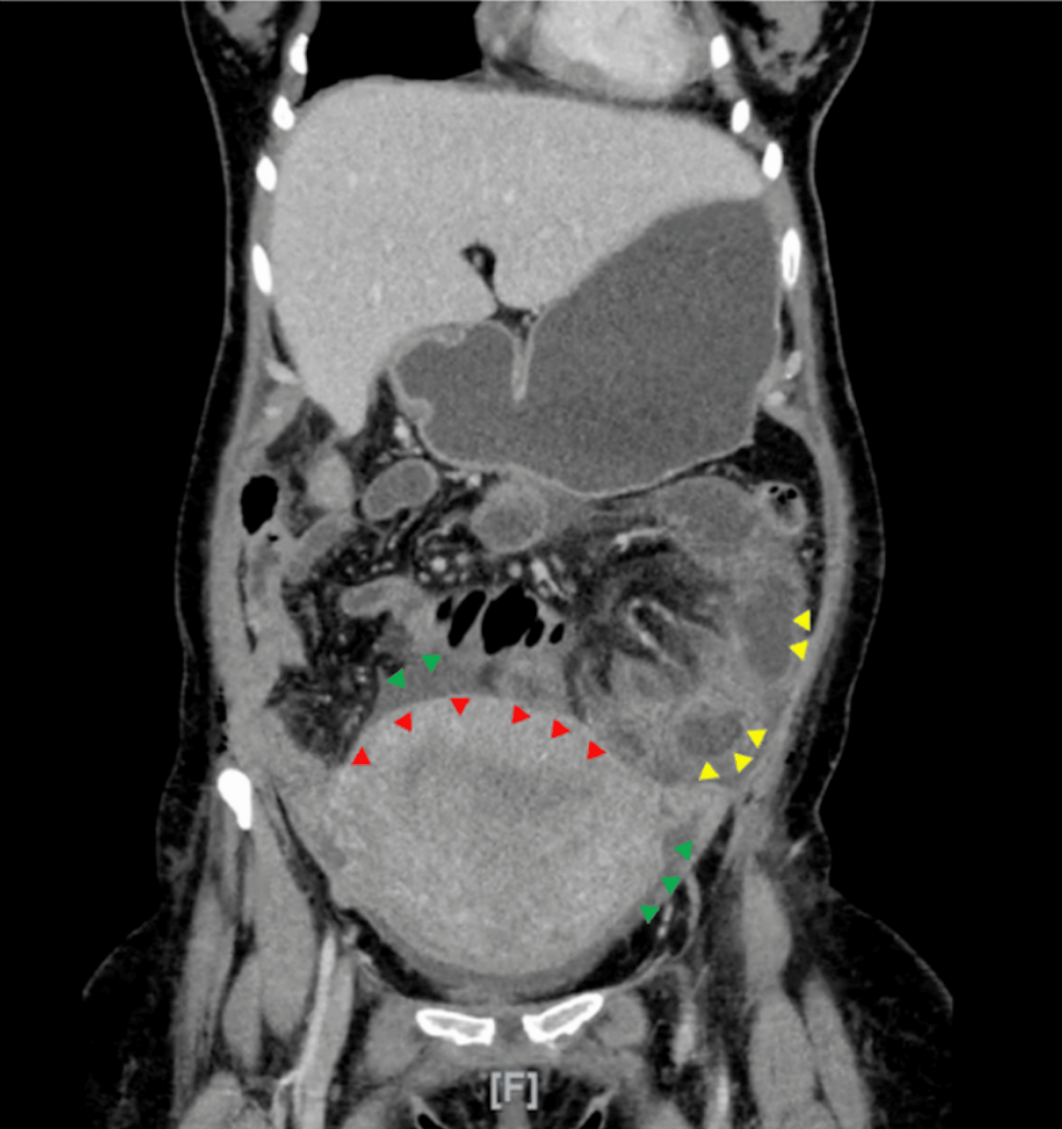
Coronal slice through contrast-enhanced CT abdomen and pelvis Performed on the day of readmission, corresponding to post-cesarean section day 4, showing red arrowheads, enlarged uterus consistent with the postpartum period; green arrowheads, pockets of free fluid in the pelvis; and yellow arrowheads, diffuse marked wall thickening and mild dilatation observed in the small bowel loops, consistent with enteritis.
